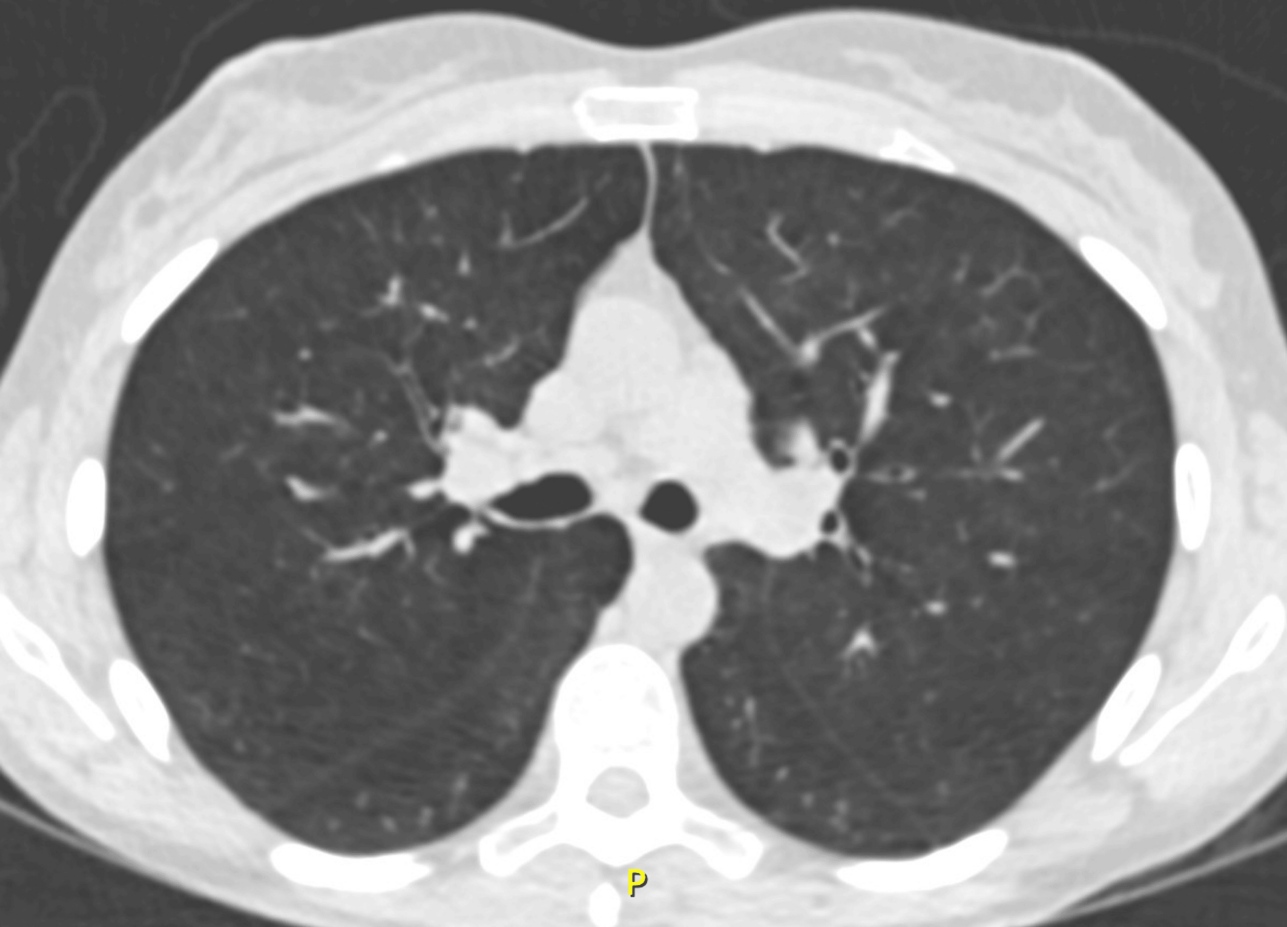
Chest CT scan showing diffuse emphysema.
